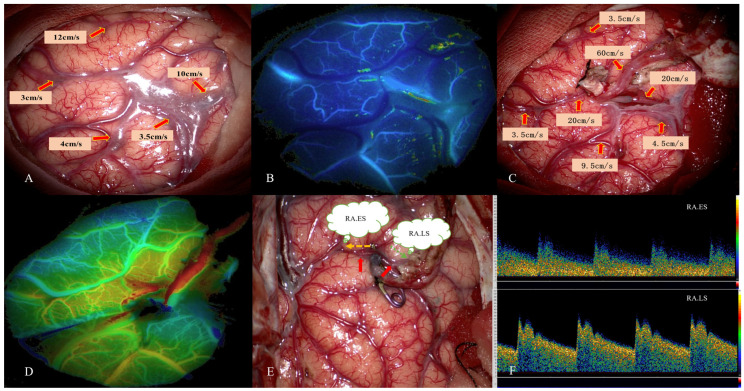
This 45-year-old female patient was diagnosed with MMD due to persistent headache. STA–MCA bypass was performed. Intraoperative images of MDU hemodynamic analysis in PSCAs were obtained. (A) Hemodynamics of PSCAs before anastomosis. RA was selected from PSCAs with MVV of 10 cm/s. (B) Preoperative blood distribution by FLOW 800 image. (C) MDU hemodynamics of PSCAs after bypass. (D) Postoperative hemodynamics evaluated by FLOW 800 image. (E) Flow direction of RA (red arrow) after bypass. The orange dotted arrow represents RA.ES, and the green represents RA.LS. (F) MDU spectrum of RA.ES_post_ and RA.LS_post_ after bypass. This patient had no postoperative CHS. MDU = microvascular doppler ultrasonography, PSCAs = para-sylvian cortical arteries. CHS = cerebral hyperperfusion syndrome. RA.ES = recipient artery entering sylvian. RA.LS = recipient artery leaving sylvian.
